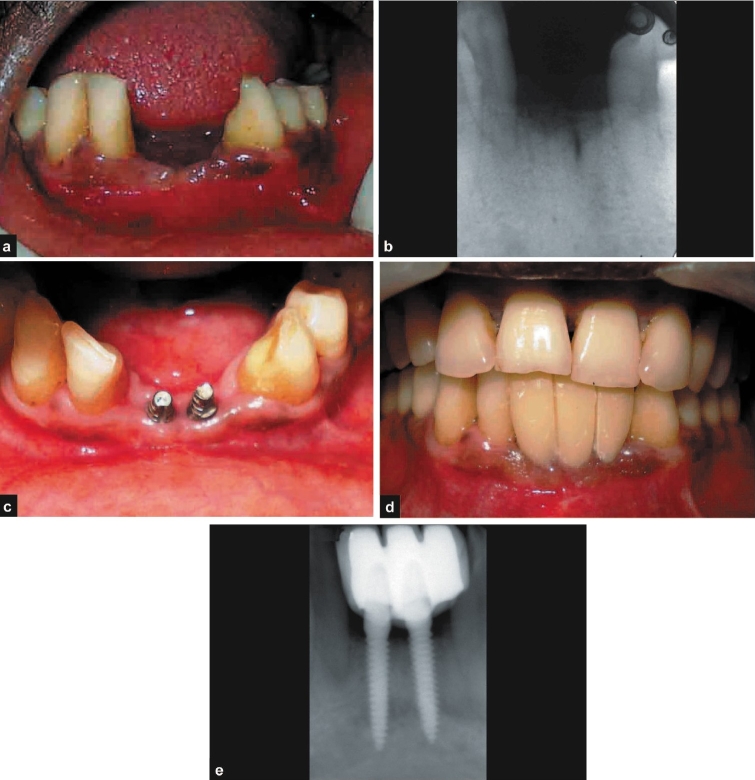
a, b) Pre-operative photograph and radiograph; c-e) post-operative photographs and radiograph
